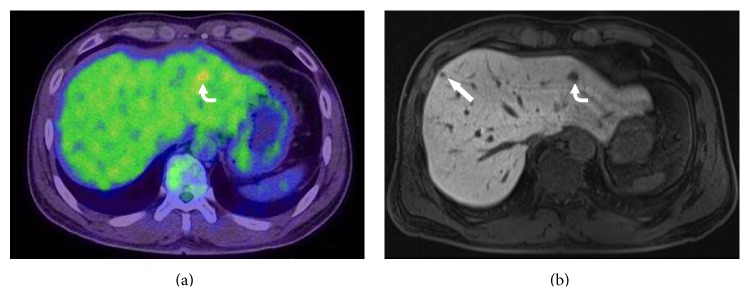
A 46-year-old man with sigmoid colon cancer, who showed one metastatic nodule on PET/CT and two metastatic nodules on liver MRI in both hepatic lobes. (a) Axial ^18^F-FDG-PET/CT image shows a focal increased uptake in left lateral section of the liver (curved arrow), but there is no definite FDG uptake in right hepatic lobe. (b) Axial image of hepatocyte-phase on liver MRI shows focal signal defects in left lateral section of the liver (curved arrow), and also in the subcapsular portion of right hepatic lobe (arrow), suggesting two metastatic nodules in both hepatic lobes.
